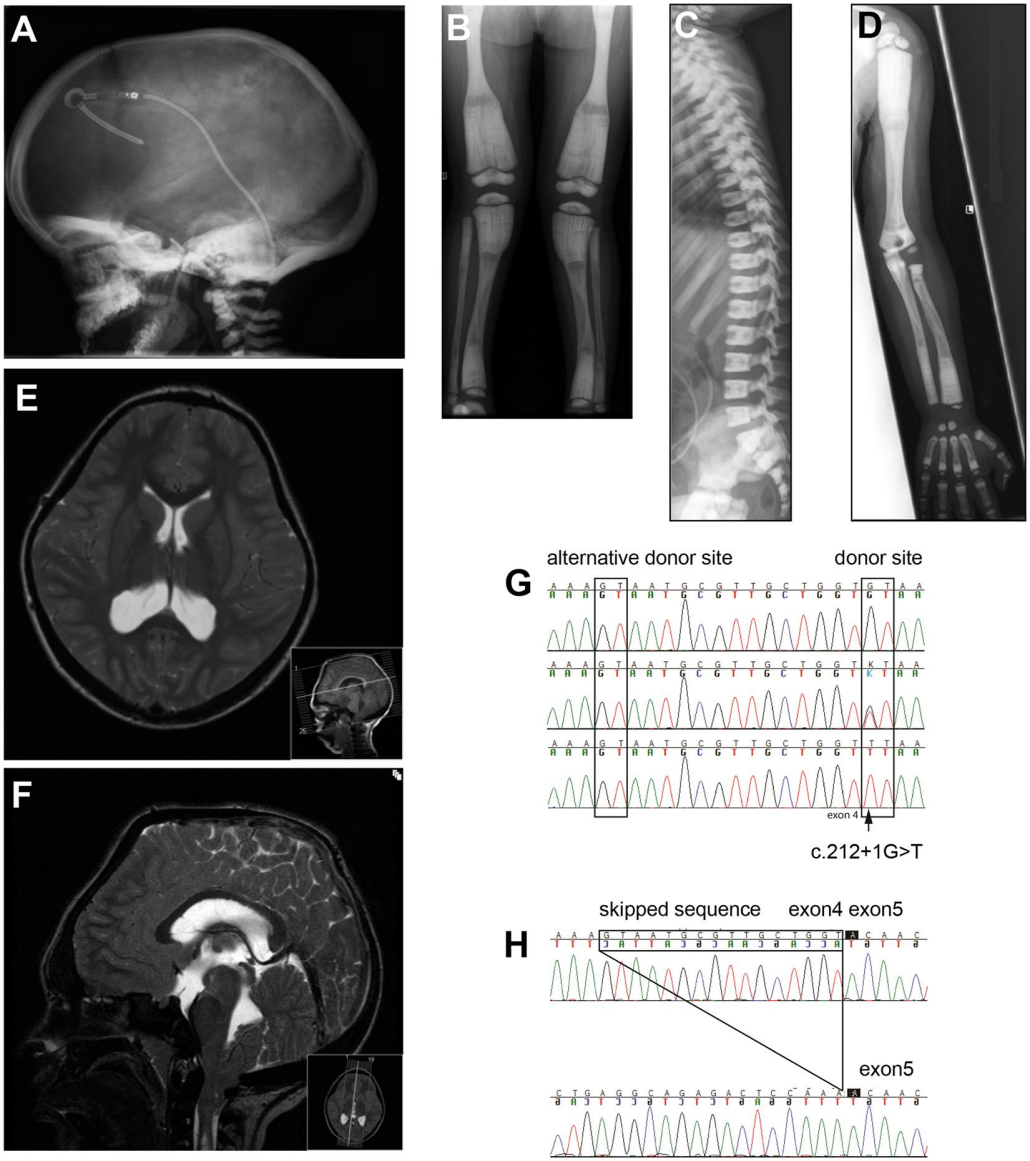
A Radiographs of subjects with the Västerbotten form of intermediate osteopetrosis, and sequencing of the *SNX10* gene. (A–D) Skeletal radiographs of a girl at the age of three years (Pt9), showing characteristic features of intermediate osteopetrosis. (A) Skull: Increased bone density of the skull base, frontal bossing and VP-shunt (B) AP view of the legs: “Erlenmeyer flask” shape of the femoral diaphysis. Longitudinal and transverse bands of lesser density in the metaphyses. Bone-within-bone pattern in the epiphyses in the knee. (C) Lateral view of the spine: Very dens endplates of the vertebral bodies, so called “sandwich vertebrae”. The ribs (seen in part) are broad and dens. (D) AP view of the left arm: No fractures or sign of rickets, “bone-within-bone” pattern of the phalanges. (E,F) Magnetic resonance imaging of the brain, and spine in an 11-year-old boy. MRI shows cerebellar tonsillar descent through the foramen magnum, a foramen magnum narrowing, and signs of brain stem compression with resulting syringo-hydromyelia. (G,H) Consequence of the c.212 + 1 G > T variant on (G) genomic level and (H) transcript level. (G) Sequencing of DNA from a control (top), a heterozygous carrier (middle) and a homozygous patient (bottom) for the c.212 + 1 G > T variant. The variant changes the donor splice site at the 5′ end of intron 4 from GT to TT. This leads to a predicted use of an alternative donor splice site 16 nucleotides upstream of intron 4. (H) Sequencing of cDNA from a control (top) and a patient (bottom) confirms the use of an alternative splice site and the skipping of 16 bp leading to a frame shift and a premature stop codon (p.S66Nfs * 15) at the protein level.
